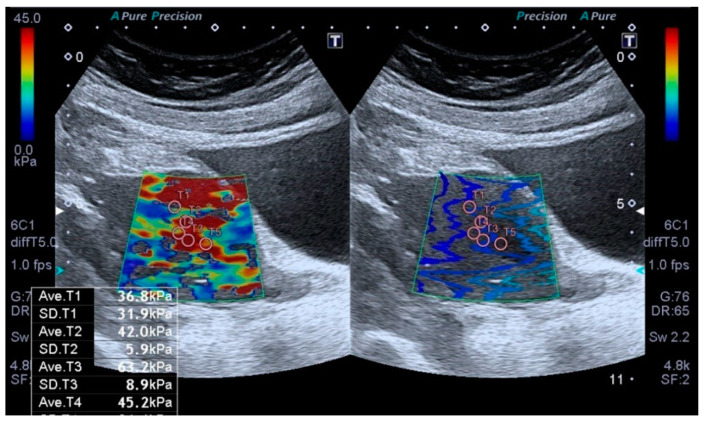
Transabdominal ultrasound examination of the endometrium, including shear wave elastography (SWE). Placement of sample points of the region of interest (ROI) in the endometrial lesion for SWE measurements.
